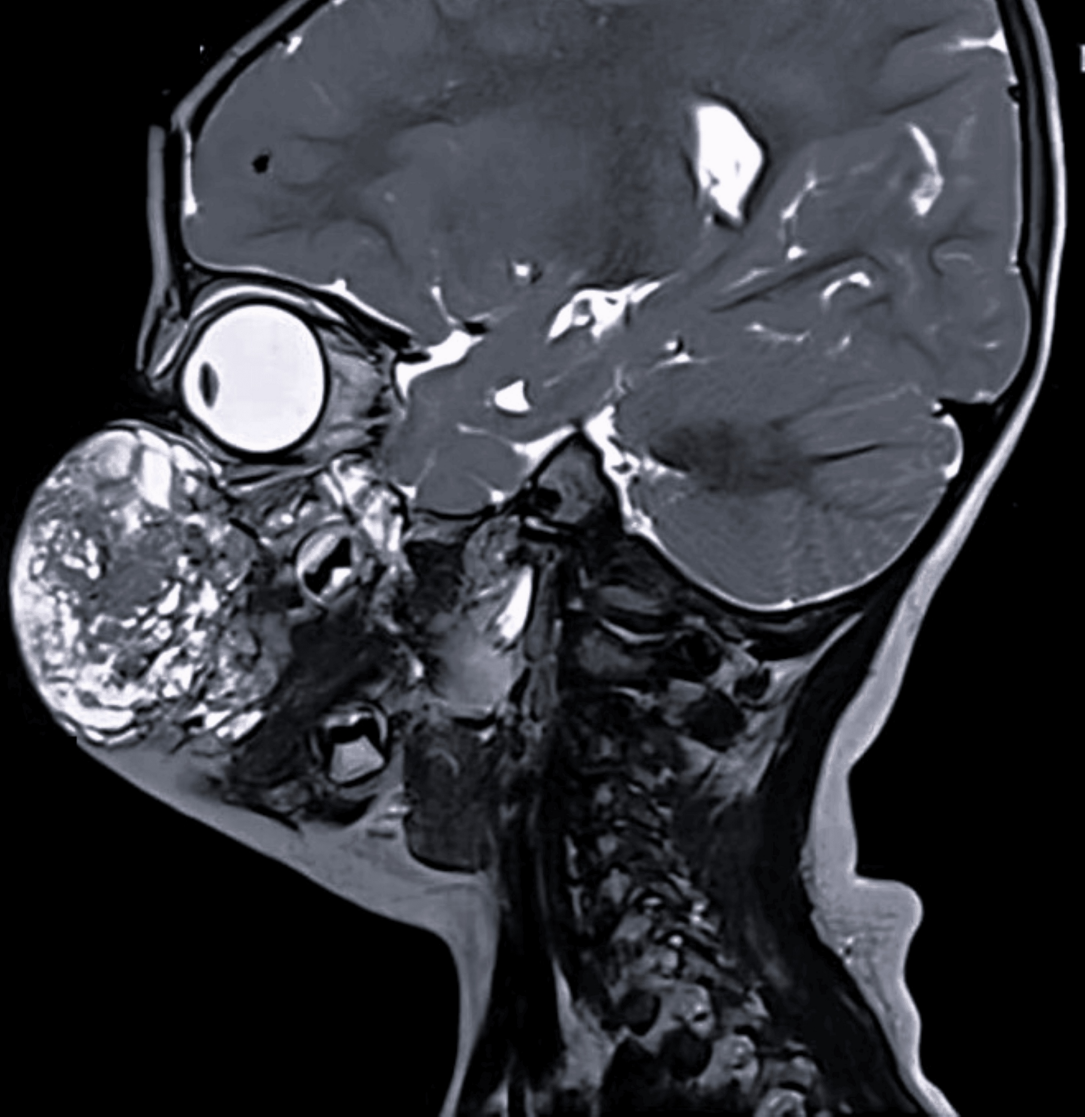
MRI scan showing a lesion of size 3.2 × 5.6 × 5.2 cm involving skin and subcutaneous tissue of the right side of the cheek extending superiorly on the posterior aspect of zygomatic bones, retromaxillary space, up to the level of sphenopalatine foramina and medially up to the medial canthus of the right eye MRI: magnetic resonance imaging
